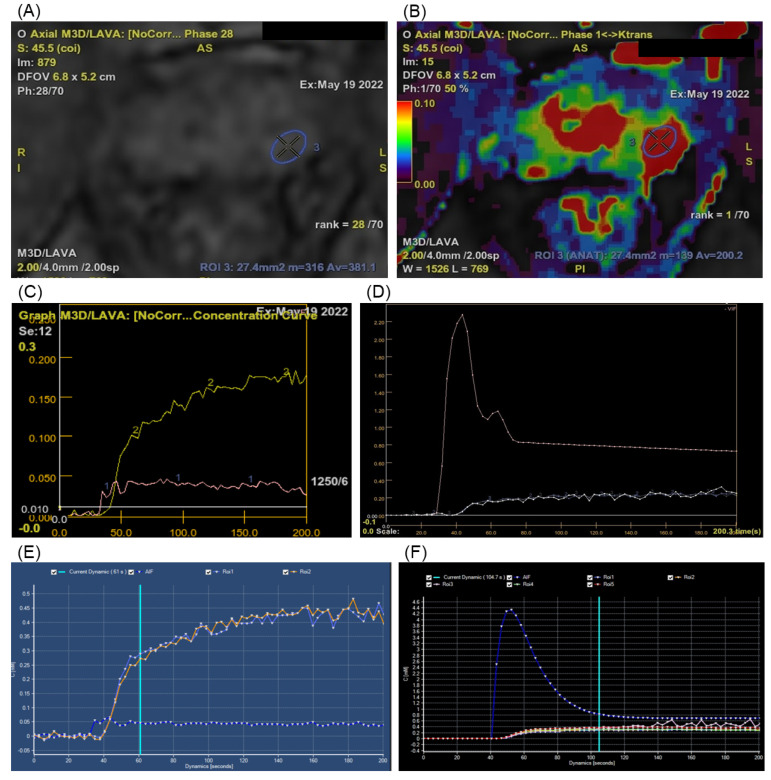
Comparison of arterial input functions (AIF) time-concentration curves obtained from two solutions (A to D, GenIQ; E-F, ISP) in a 70-year-old man with prostate cancer in the left middle peripheral zone (Gleason score 7). Multiple ROIs from tumor are presented in various colors. (A) Axial DCE-MRI image on GenIQ showing placement of a region of interest (ROI) within the index tumor. (B) Corresponding K^trans^ (volume transfer constant) map with the tumor ROI overlaid. (C) Individual AIF in GenIQ, derived from manually placed external iliac arterial ROI; AIF graph is in orange, and tumor graph is in yellow. The calculated K^trans^ was 0.2/min. (D) Population-based AIF in GenIQ, with an arterial peak of approximately 2.3 mM. The calculated K^trans^ was 0.2/min. (E) Individual AIF in IntelliSpace Portal (ISP); AIF graph is in blue; tumor graphs are from ROI 1 and 2. The average calculated K^trans^ was 10.1/min. (F) ISP population-based medium preset AIF, showing arterial peak of approximately 4.4 mM. The calculated K^trans^ was 0.1/min. These differences in AIF type and curve profile may contribute to variability in estimated perfusion parameters.
